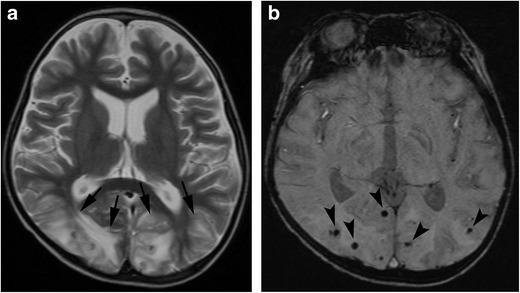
Posterior reversible encephalopathy syndrome. (PRES). A 5-year-old boy with acute lymphocytic leukaemia, who received bone marrow transplantation, developed lethargy during administration of tacrolimus. a A T2-weighted image shows hyperintensities in the occiptal lobes (*arrows*). b A susceptibility-weighted image (SWI) shows punctate signal loss in these lesions, suggestive of microhaemorrhage (*arrowheads*)
